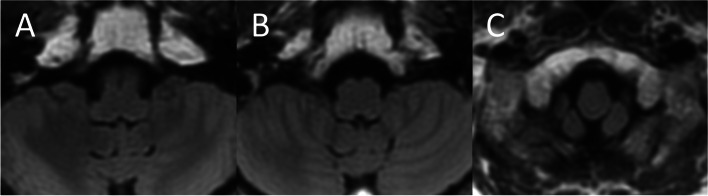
Classification of the lesion in the rostro-caudal direction on brain magnetic resonance imaging. a Upper medulla: the level with an inferior cerebellar peduncle bulging outward. b Middle medulla: the level with an outward bulging due to the inferior olivary nucleus. c Lower medulla: the level presenting a round shape, and without an outward bulging due to the inferior olivary nucleus. These images are the authors’ original work
